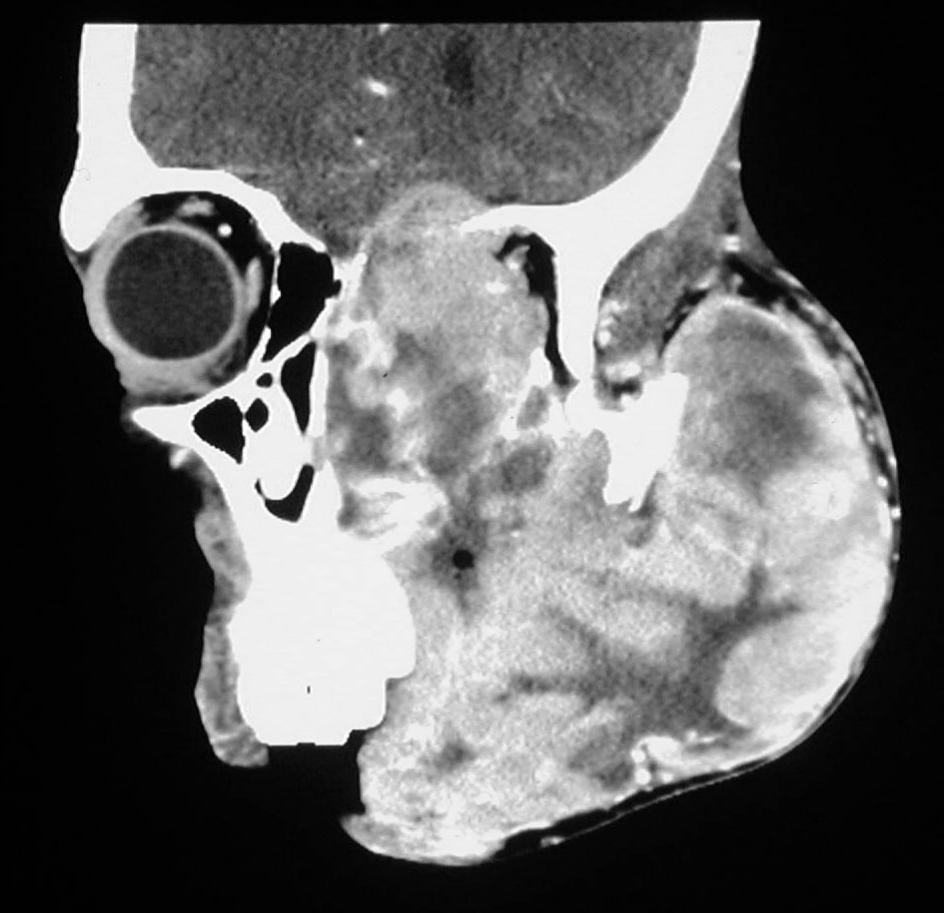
Sagittal CT cut showing the extensive destruction of the left maxilla, and the left paranasal tissues. The left orbit is filled with tumorous tissue.
